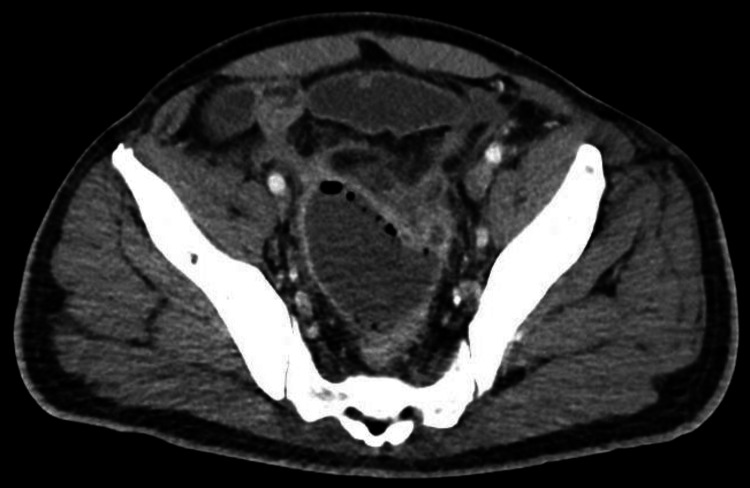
Axial contrast-enhanced CT image demonstrating interval enlargement of the central pelvic fluid collection, now measuring approximately 7.5 × 6.4 cm, with associated inflammatory changes, findings consistent with progression of a pelvic abscess.
